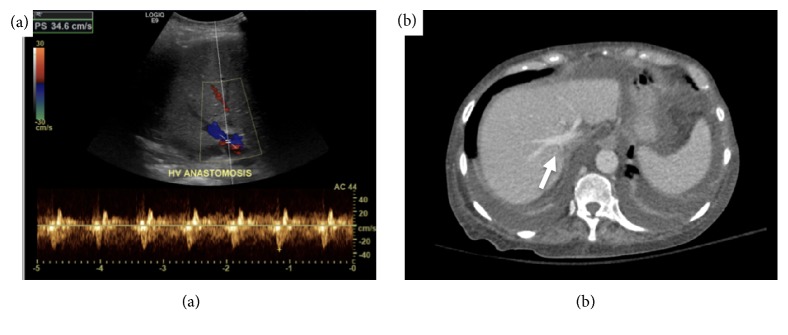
Posttransplant imaging reveals no sequelae of removal of retained CVC.* Panel (a): liver ultrasound with Doppler showing normal hepatic venous anastomosis waveforms and velocities. Panel (b): axial contrasted CT scan showing patent, normal appearing hepatic venous anastomosis (arrow).
